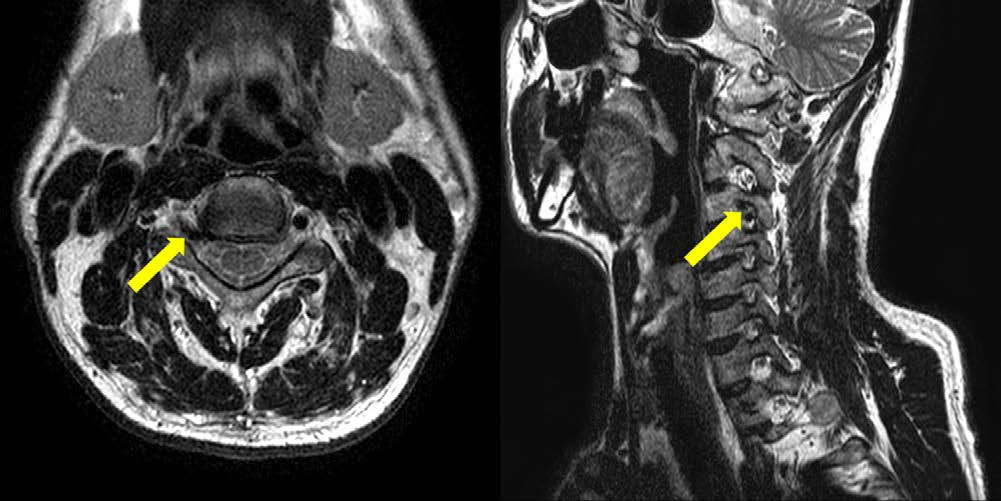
T2-weighted magnetic resonance imaging (MRI) scan of the cervical spine. Axial section at C3/4 (left) and oblique section (right). The axial section shows compression of the nerve root (yellow arrow) by the vertebral artery loop around the foraminal zone at the right C3/4 level. The oblique section shows a vertebral artery flow void instead of a nerve root at the right C3/4 level (yellow arrow).
